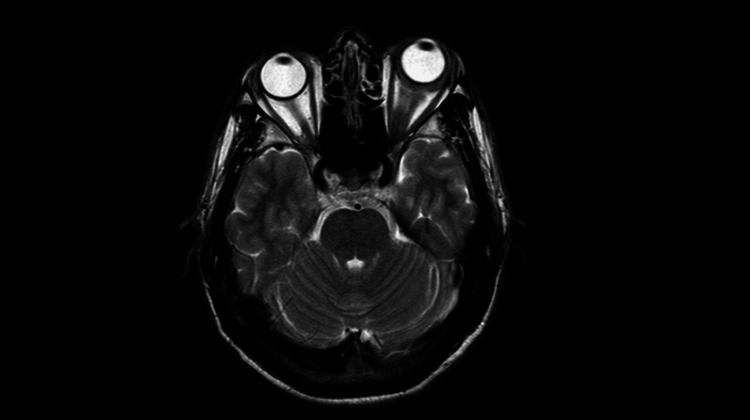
Axial T2-weighted magnetic resonance imaging (MRI) sequence showing structural changes in the left optic nerve, with no visible abnormalities in the brain parenchyma.
